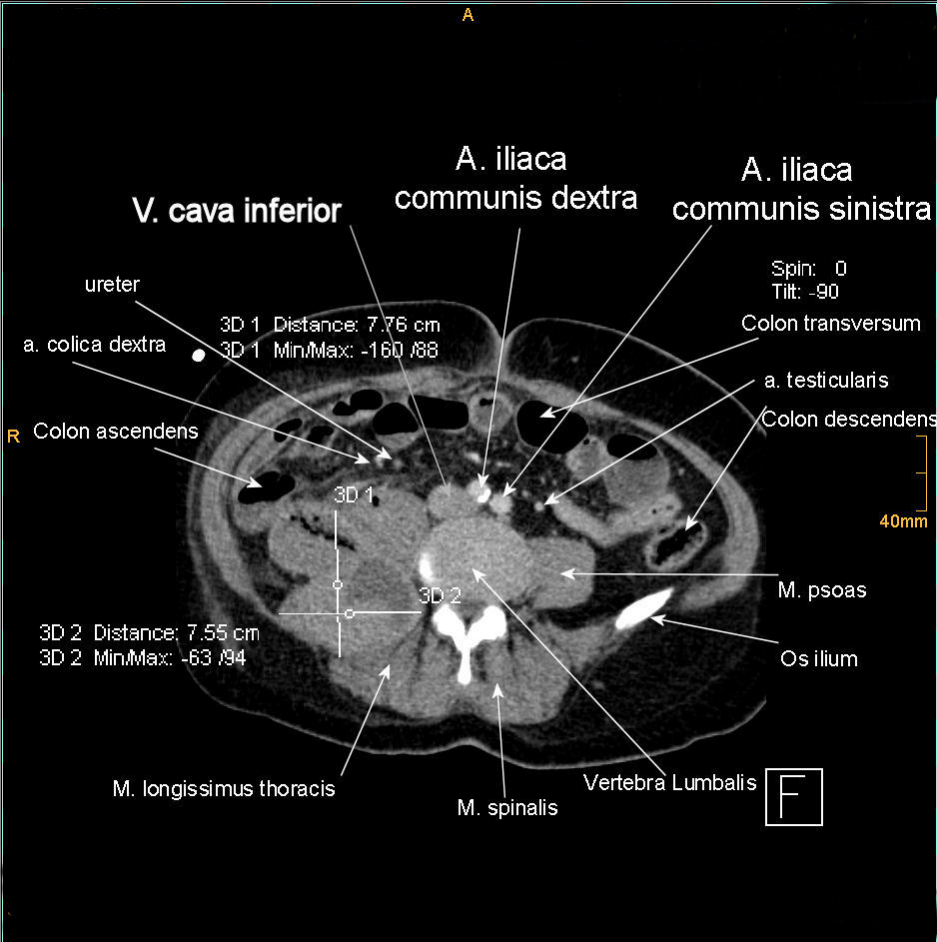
2^nd ^case. CT - axial plan of the hematoma shifting the right ureter to the middle line.
